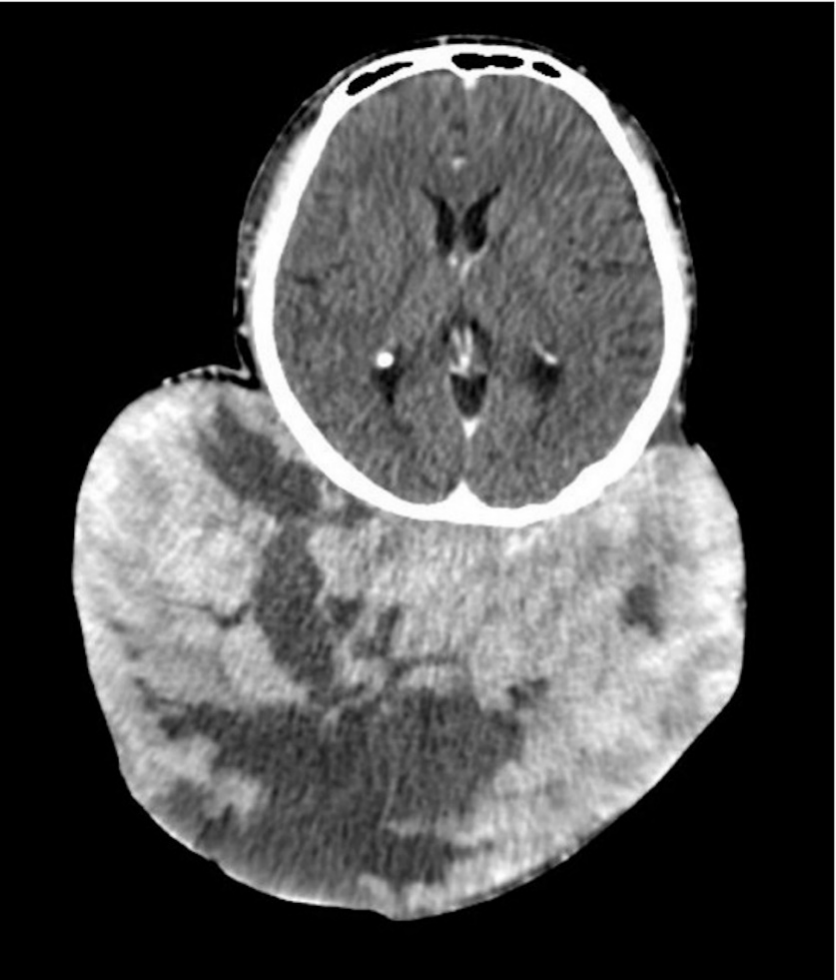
CT scan of brain with contrast (axial view).
